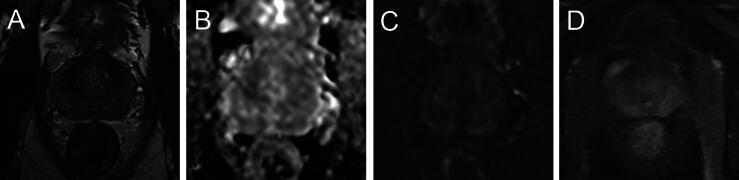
For 50-yr-old men, PSA 0.39 ng/ml (PSAD 0.01 ng/ml/cm^3^). Multiparametric MRI with (A) severe diffuse but no focal T2w changes in the peripheral zone (PZ), (B) no focal changes on ADC map or (C) high b-value images, and (D) slight symmetric enhancement on DCE in PZ, PI-RADS 3, and PI-QUAL 4. ADC = apparent diffusion coefficient; DCE = dynamic contrast enhanced imaging; MRI = magnetic resonance imaging; PI-QUAL = Prostate Imaging Quality; PI-RADS = Prostate Imaging Reporting and Data System; PSA = prostate-specific antigen; PSAD = PSA density; PZ = peripheral zone; T2w = T2 weighted.
